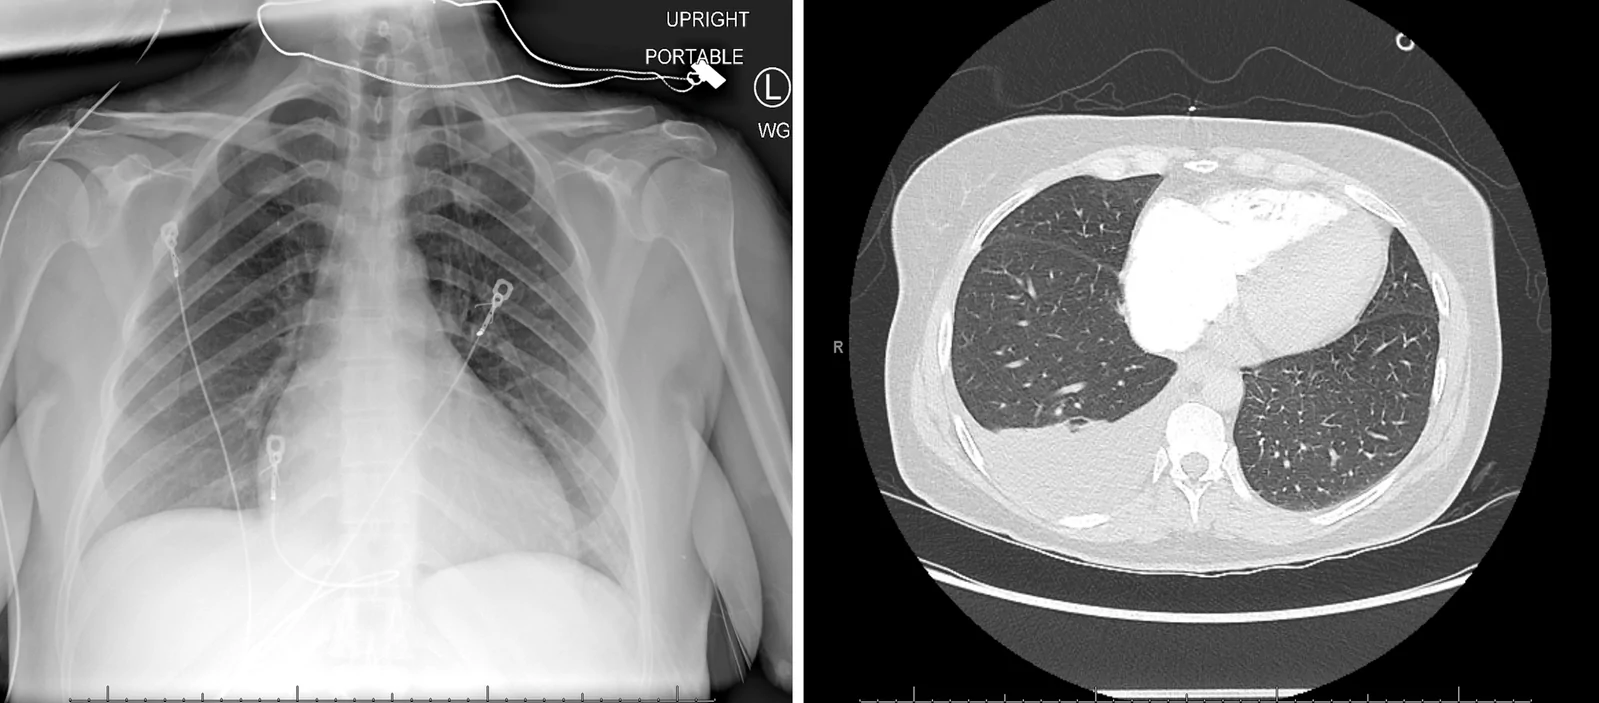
Chest X-Ray With Small Right-Sided Pleural Effusion Computed tomography pulmonary embolism with large right-sided effusion not seen in chest x-ray and minimal left-sided effusion.
